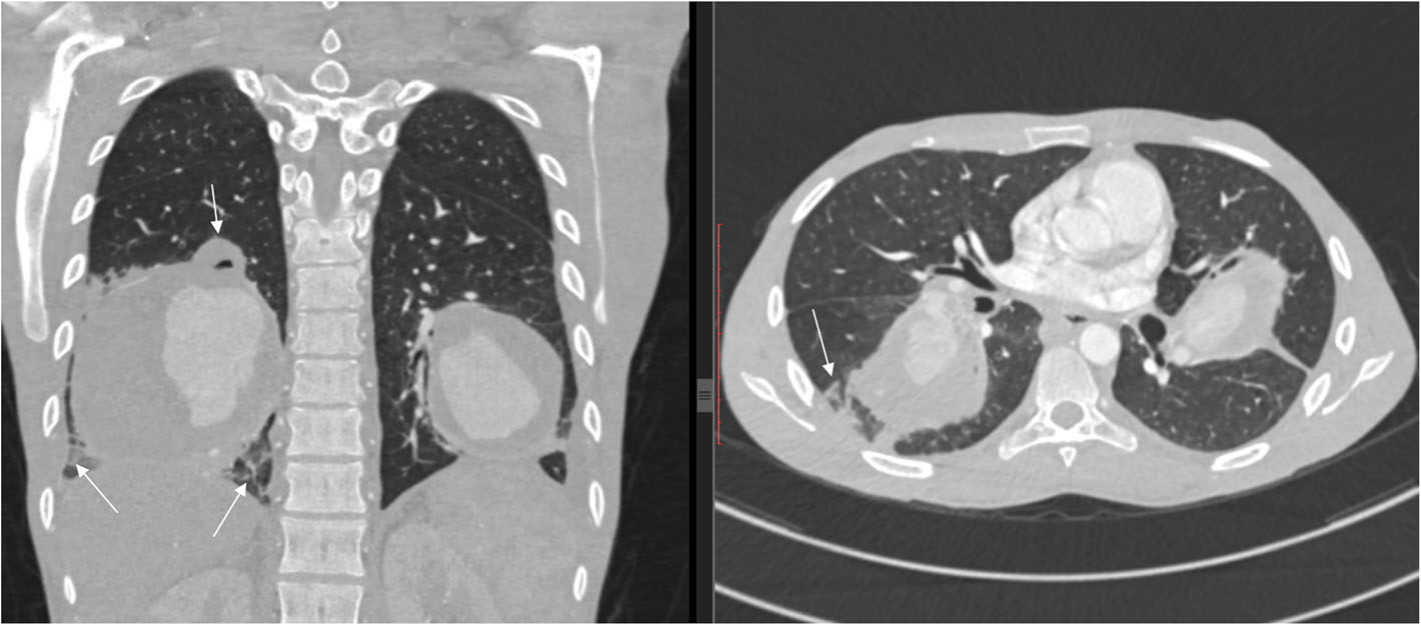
Computed tomography chest scan (coronal and axial views) showing bilateral pulmonary artery pseudoaneurysms with adjacent cystic changes (*arrows*), consolidations and tree-in-bud appearance
